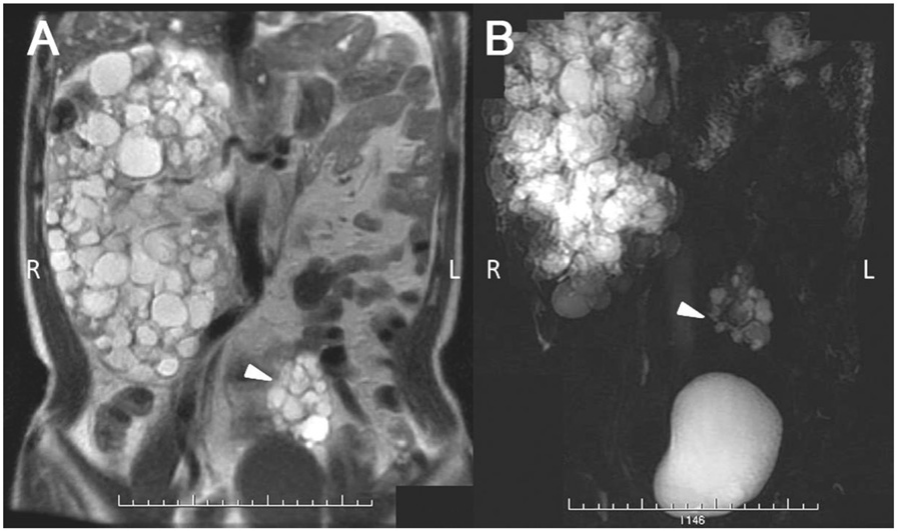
MRI scans showing a solitary right polycystic kidney with contralateral MCDK in Case 2 patient. (Triangle): left ectopic MCDK.
